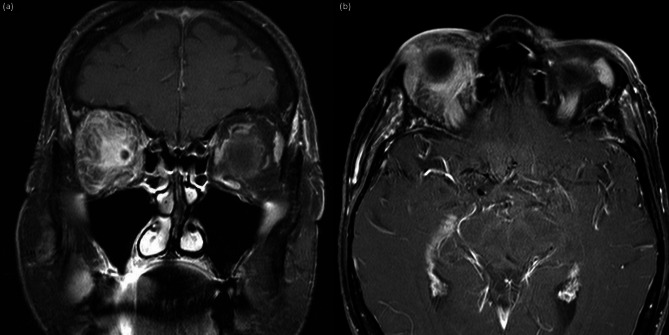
T1-weighted coronary (a) and transverse (b) MRI scan of the orbit, performed with Gadolinium contrast during the fourth episode of orbital inflammation. Notice significant enhancement and infiltration in both the preseptal and postseptal regions, including the lacrimal gland, of the right eye.
